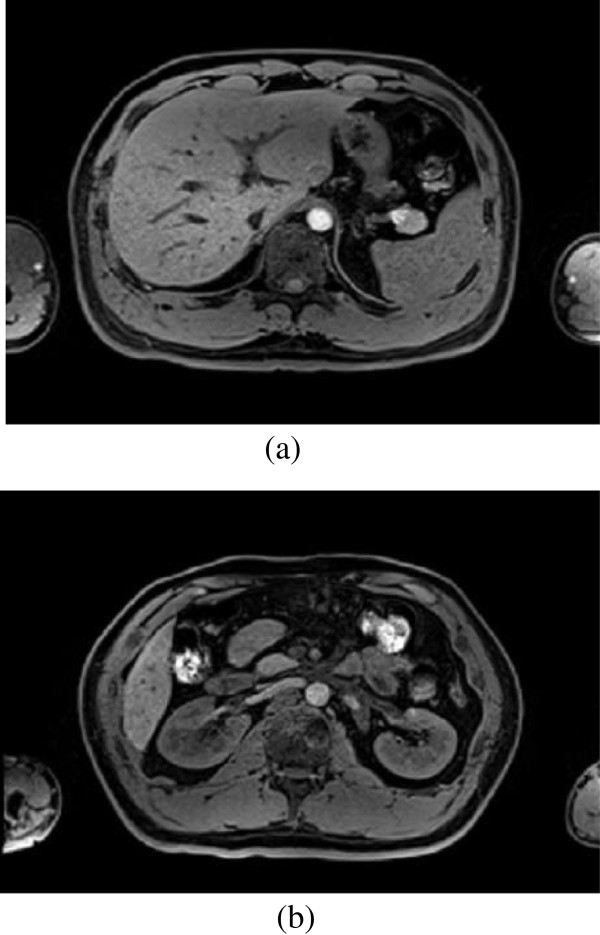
Original image, (a)the abdomen MR image of liver; (b)the abdomen MR image of kidney.
